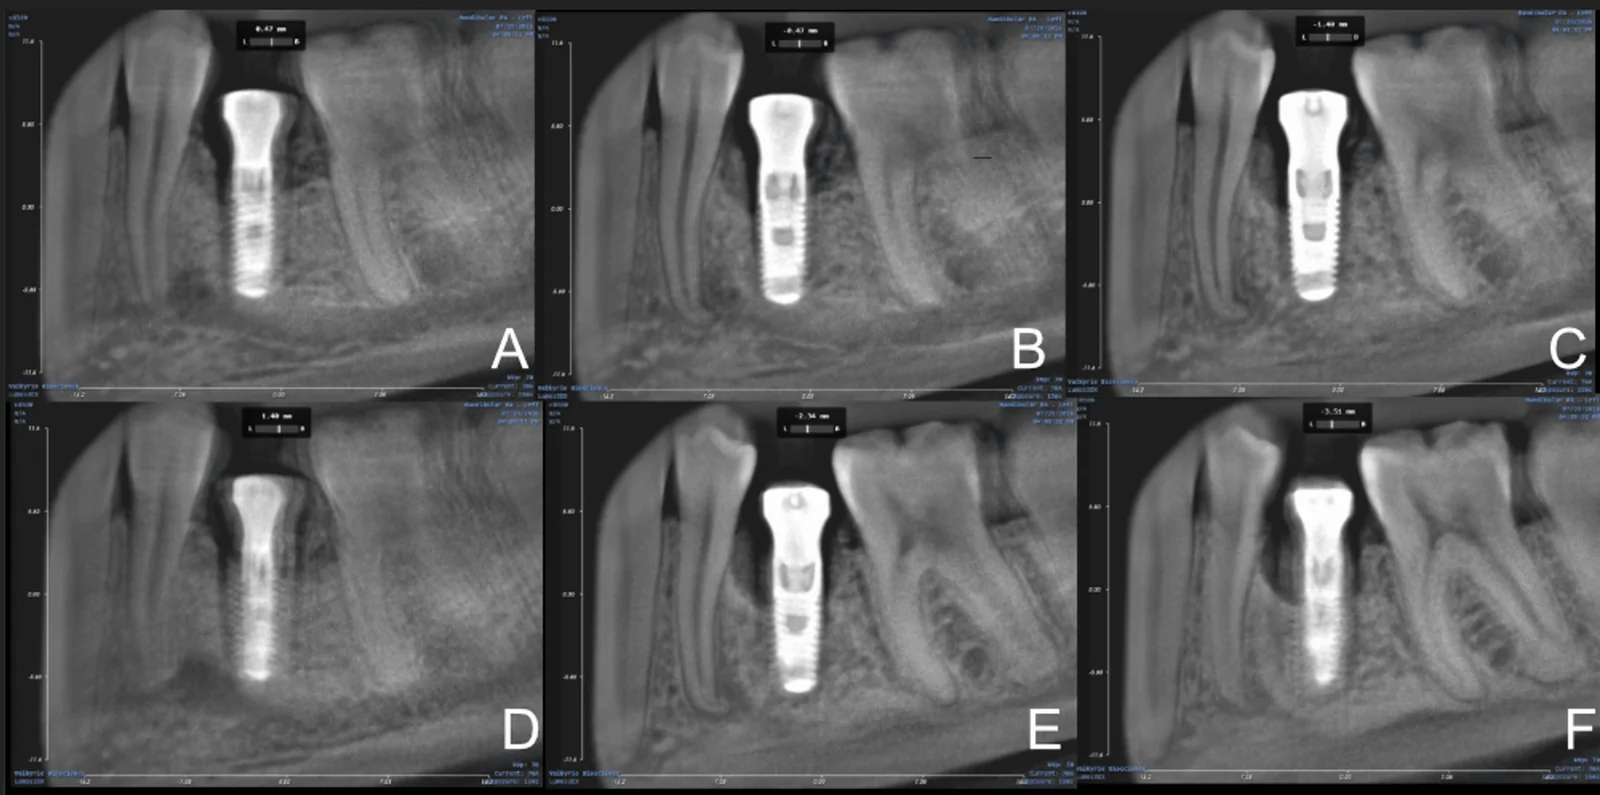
Lumos 3DX tomosynthetic images of the same implant site demonstrating multiple sectional views (A = -0.41, B = -0.49, C = -1.03, D = -1.45, E = -2.54, and F = -3.51) through the implant and surrounding bone, providing information not available on the conventional 2D radiograph (Figure 7).
